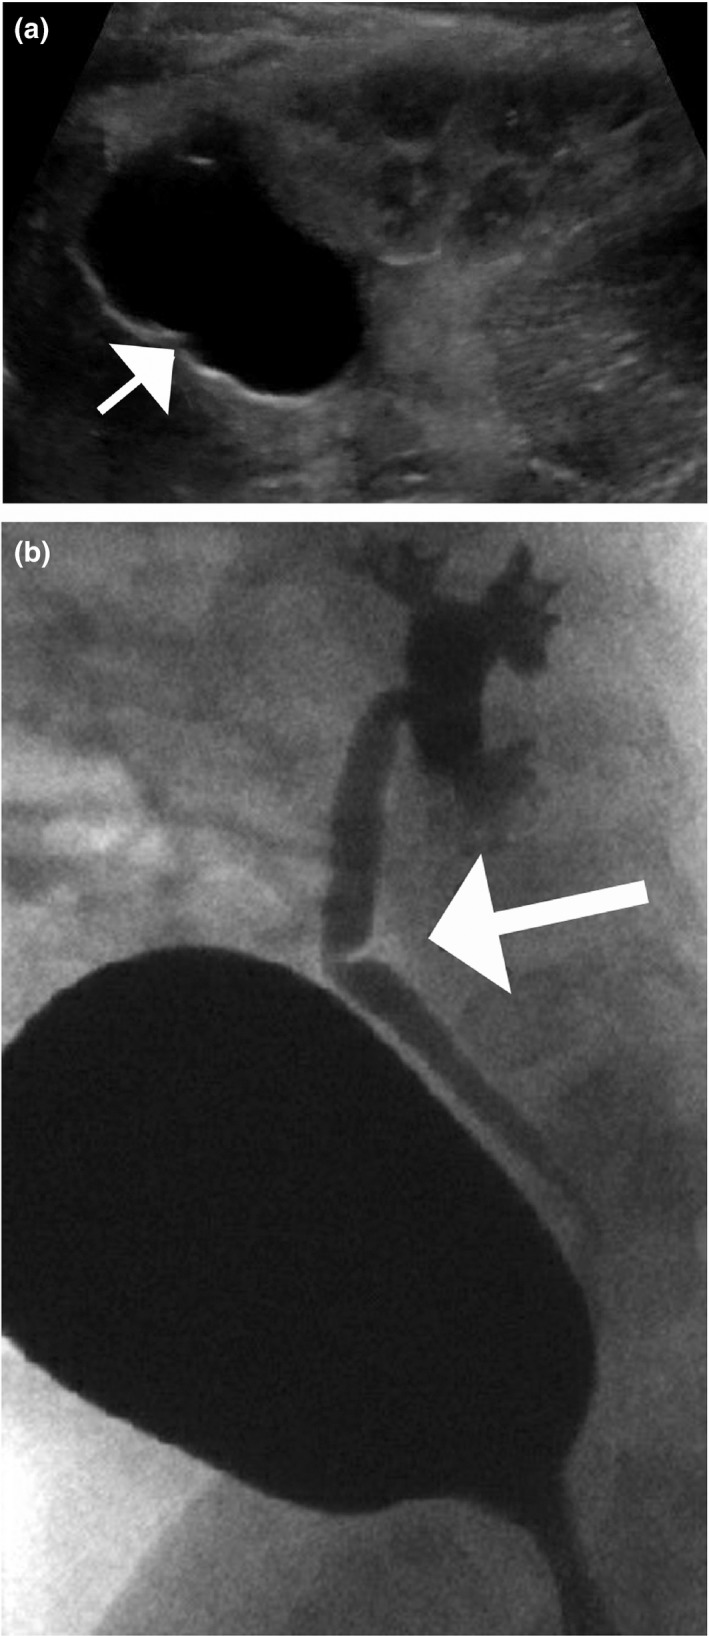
(a) A renal and bladder ultrasound demonstrated a cyst (arrow) on the superior aspect of the left kidney measuring 2.4 cm. (b) A voiding cystourethrogram revealed a ureteral valve in the middle aspect of the left ureter (arrow)
